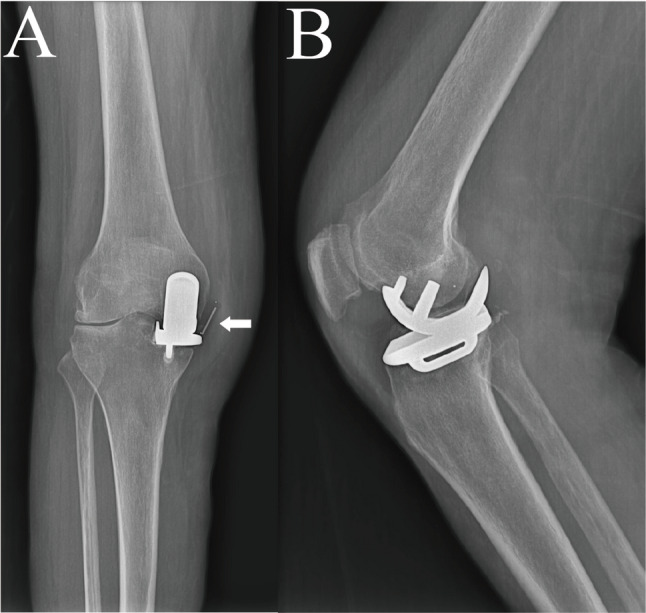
Postoperative radiographs of the right knee following unicompartmental knee arthroplasty. A Anteroposterior view: Displacement of the polyethylene bearing into the medial joint space is indicated by the white arrow. Noticeable radiolucent lines are observed along the periphery of the tibial component. B Lateral view: Abnormal positioning of the implant is evident
